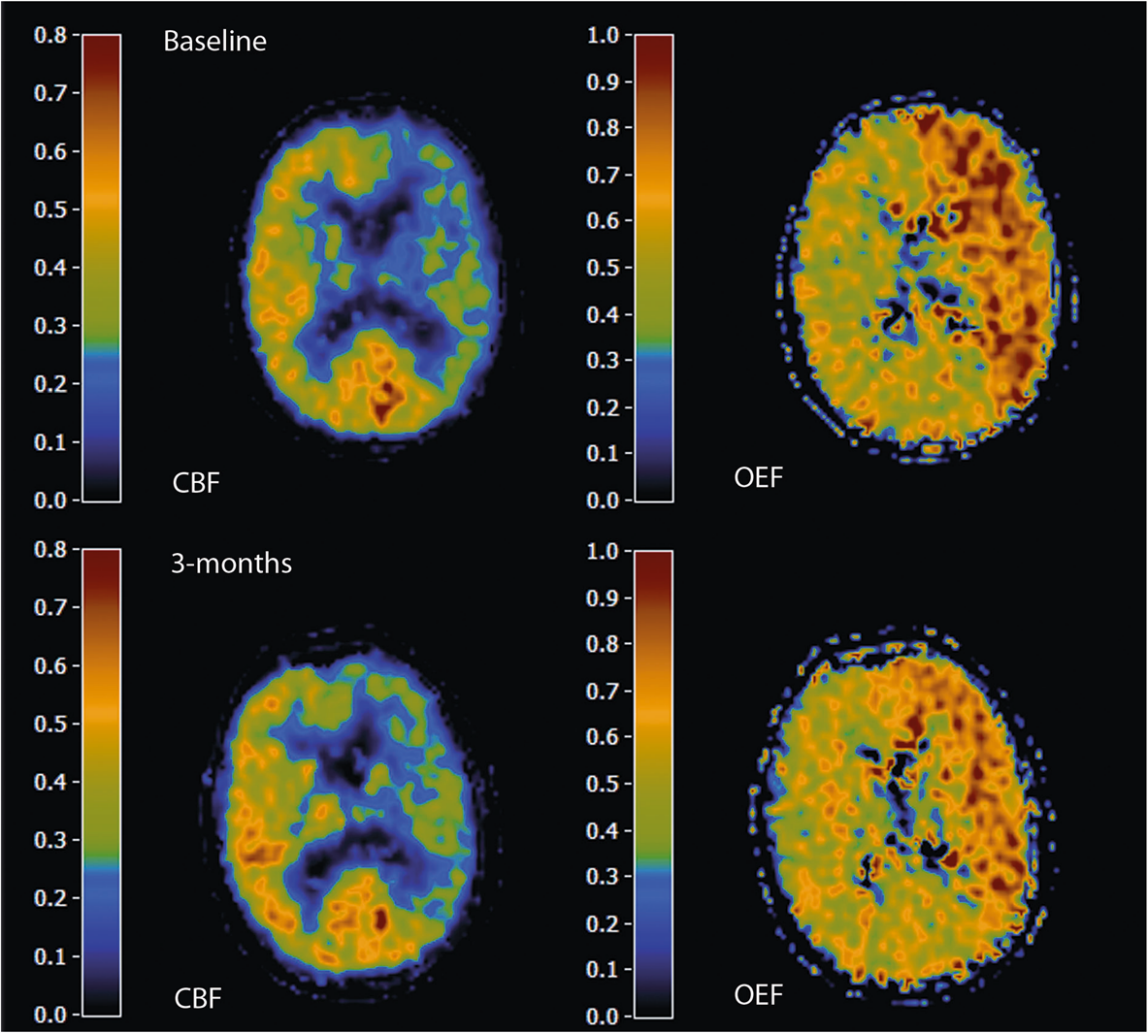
Parametric images of a patient with stage 2 haemodynamic failure. This 69-year-old man presented with a minor ischaemic stroke (without infarction on his MRI) and recurrent TIAs from the left hemisphere associated with a left ICA occlusion. The baseline PET study showed decreased cerebral blood flow (CBF) and increased oxygen extraction fraction (OEF) in the territories of both anterior and middle cerebral artery of the left hemisphere. This patient was randomised for tapering of antihypertensive medication. The PET study after 3 months showed a slight improvement in CBF and OEF. However, he still had recurrent TIAs in the presence of stage 2 haemodynamic failure.
